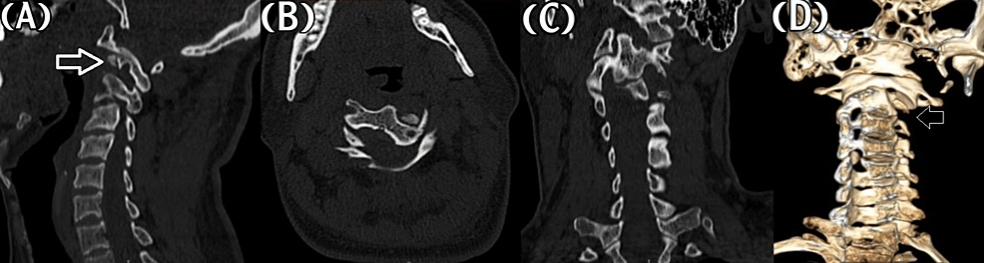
Preoperative CT of the cervical spine Sagittal (a), axial (b), and coronal CT (c), and CT with 3D (d) bone window. The CT images of the cervical spine demonstrate the left atlantoaxial rotatory subluxation (arrows), where the atlas rotated on the odontoid.
